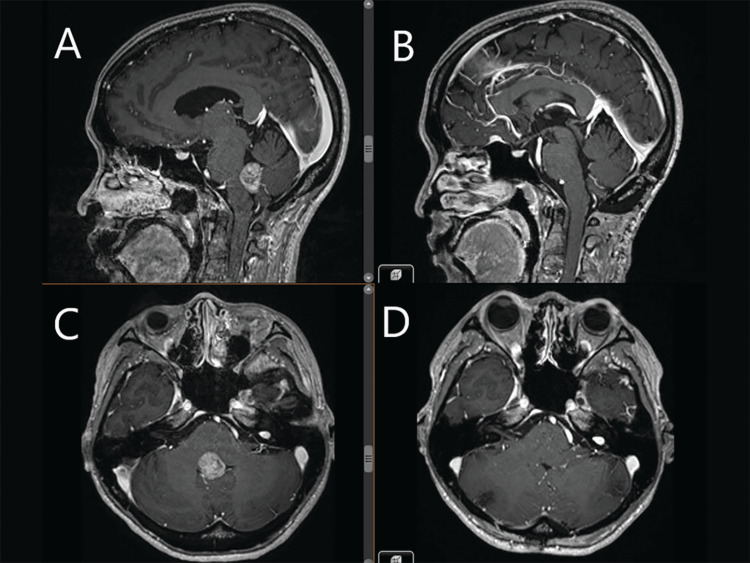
Pre- and post-operative MRI images (A) and (C) Pre-operative contrasted T1-weighted MRI (sagittal and axial) demonstrating a heterogeneously enhancing tumor obstructing the inferior half of the fourth ventricle. (B) and (D) Post-operative T1-weighted MRI (sagittal and axial) performed seven months later, demonstrating complete excision of the tumor without recurrence.
